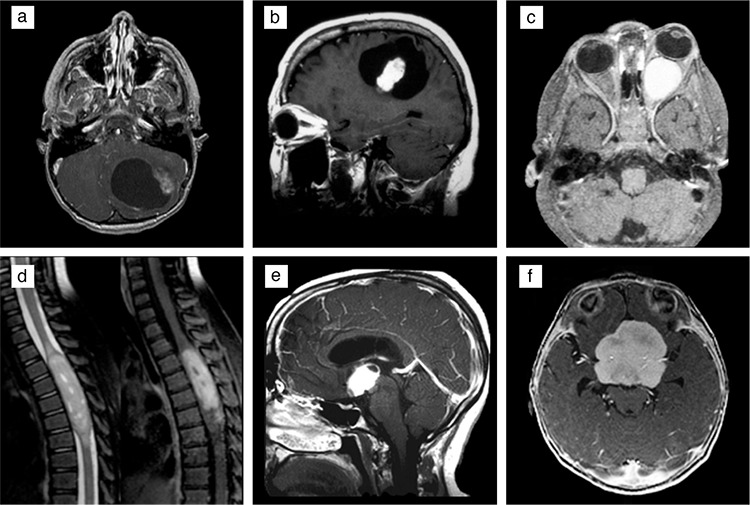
Radiologic features of pilocytic and pilomyxoid astrocytoma on magnetic resonance imaging. Pilocytic astrocytomas displaying typical circumscribed, cystic appearances with enhancing mural nodules in the (a) cerebellum and (b) supratentorial region. Expansion of the left optic nerve by a solid, enhancing pilocytic astrocytomas (c). T2-weighted and contrast-enhanced images from an intramedullary pilocytic astrocytomas involving the spinal cord (d). Mainly solid, enhancing pilocytic astrocytomas with minimal cystic component involving the hypothalamic region (e). Lobulated, solid enhancing pilomyxoid astrocytoma involving the hypothalamic region (f). All figures are contrast-enhanced T1 weighted images, with the exception of the one-dimensional T2-weighted image showing a spinal cord tumor.
